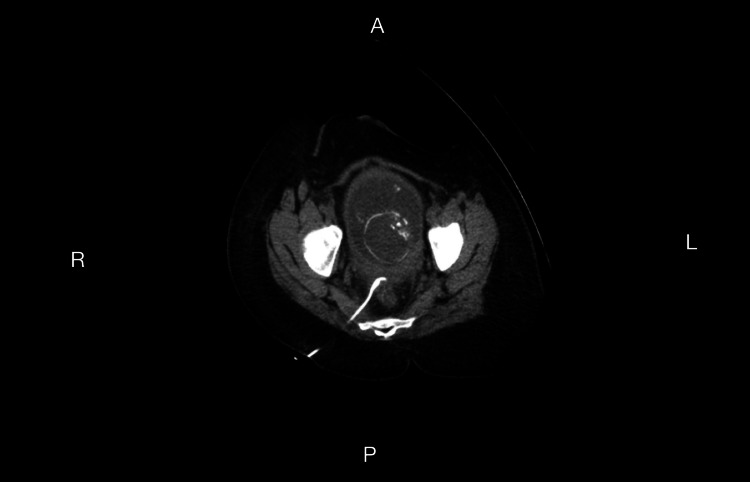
CT-Guided Drain Placement Impression: CT-guided right 8 French transgluteal drain placement into rectouterine pouch fluid collection.
